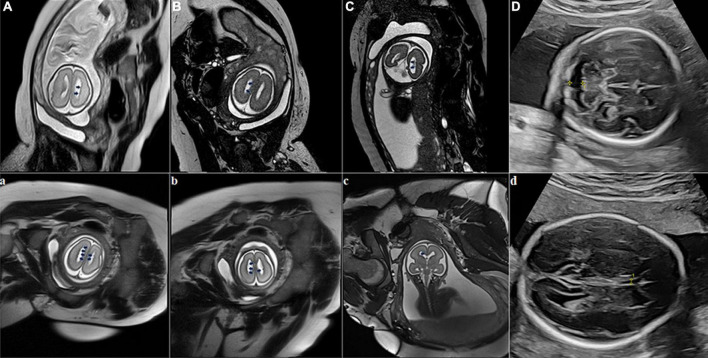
Fetal brain MRI at two fetuses of the pregnant woman. Fetus 1 (A–D), scanning at 27 + 1 weeks of gestation with bilateral subependymal heterotopia (SEH). Axial (1A, 1B) and coronal (1C) fetal MRI images show that bilateral ventricles are not dilated, ependyma is irregular, and multiple abnormal signal nodules can be seen. The T2-weighted sequence shows nodules in the ventricular wall similar to gray matter signals (black arrows). Fetal sonogram (D) Axial image, obtained at 26 weeks of gestation through the cerebellar level, shows the widened posterior cranial fossa (yellow measurement arrow); Fetus 2 (a–d), scanning at 25 + 2 weeks of gestation with bilateral SEH. Axial (1a, 1b) and coronal (1c) T2-weighted sequences at the level of the lateral ventricles show nodules in the ventricular wall similar to gray matter signals (black arrows). Fetal sonogram (1d) Axial image, obtained at 24 weeks of gestation through the double top diameter layer, shows the narrowing of the transparent compartment cavity (yellow measurement arrow).
